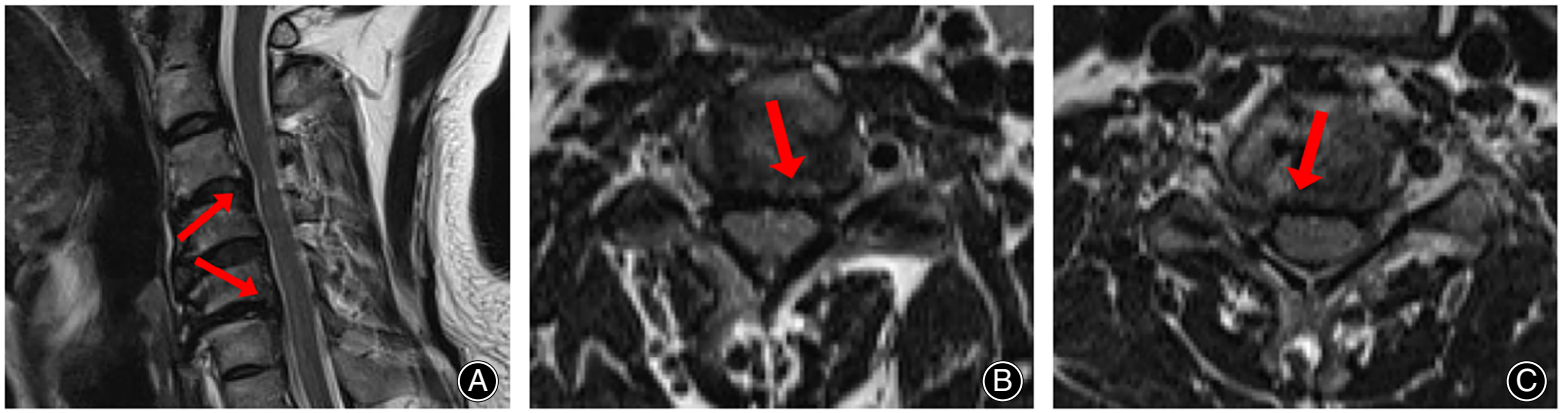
Follow‐up T2‐weighted MRI images. Sagittal (A) and transverse views (B, C) showed that the spinal cord of 3/4 and 5/6 of the neck was not compressed, and the decompression was adequate (arrow).
